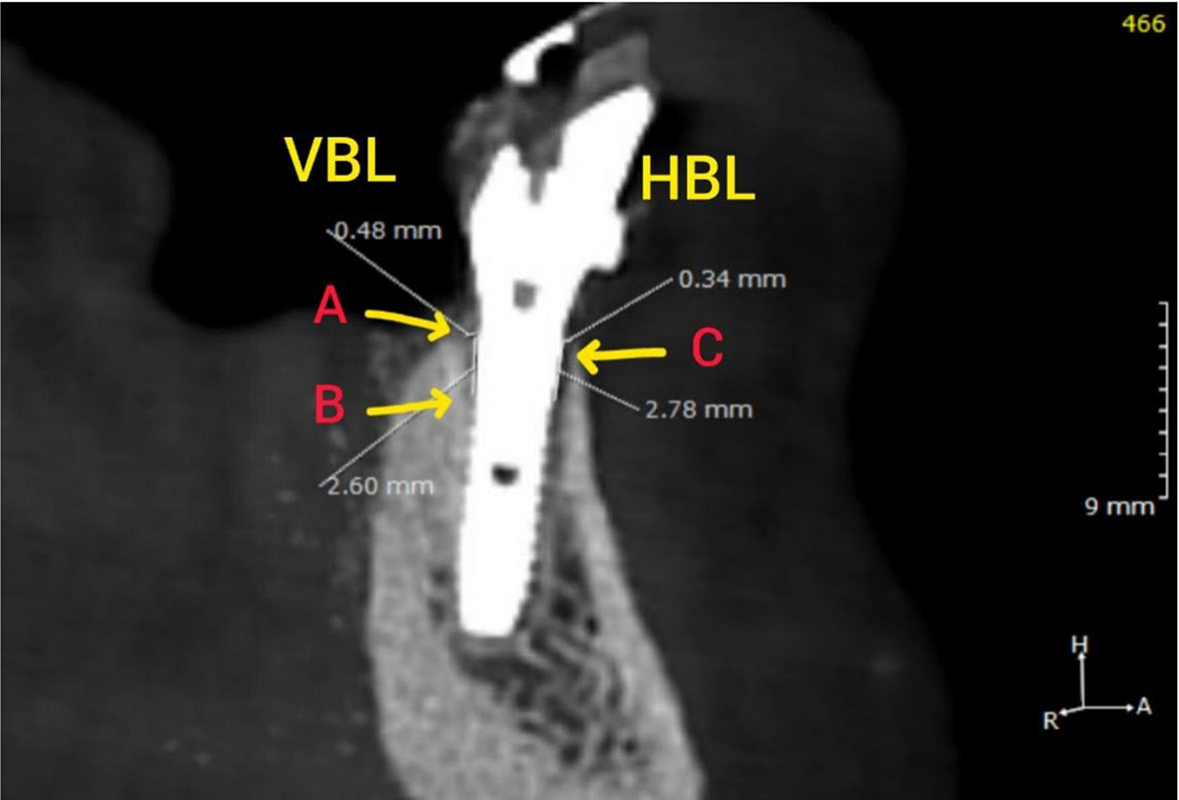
Measurements of circumferential peri-implant bone level on CBCT
